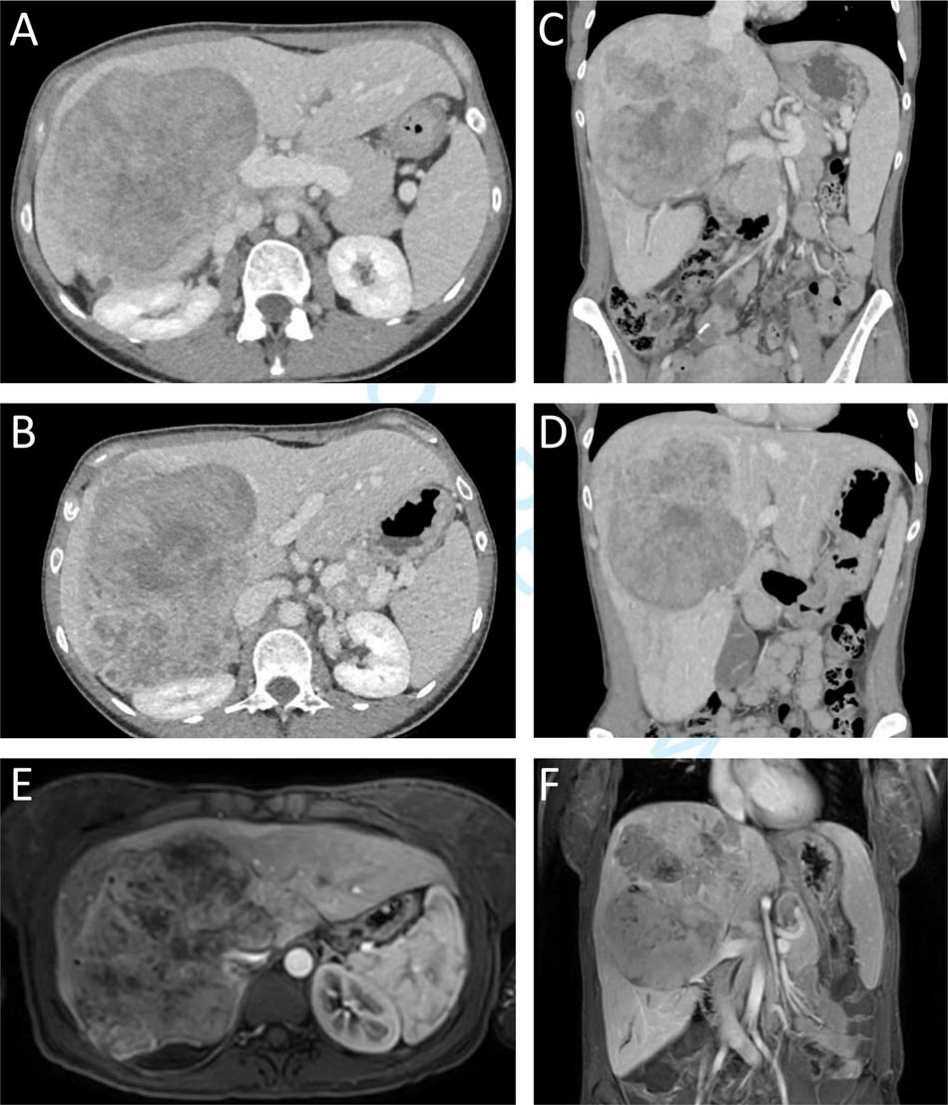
Preoperative CT and MR imaging. Axial (A + B) and coronal (C + D) CT images depicting a large mass replacing the right liver lobe, involving the retrohepratic IVC and compressing the right kidney. The portal bifurcation is free of tumour and the left portal vein is patent. (E + F) MR images depicting the large lesion occupying the right liver lobe and involving the retrohepatic IVC.
